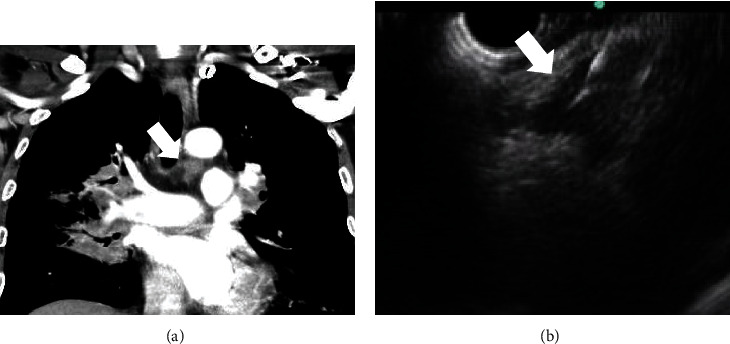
A 53-year-old male patient. (a) Left lower paratracheal lymphadenopathy (arrow) on CT scan. (b) On EUS view, triangle-shaped hypoechoic lymph node (arrow) was observed in station 4L. The EUS-guided biopsy was performed. CT: computed tomography; EUS: endoscopic ultrasound.
